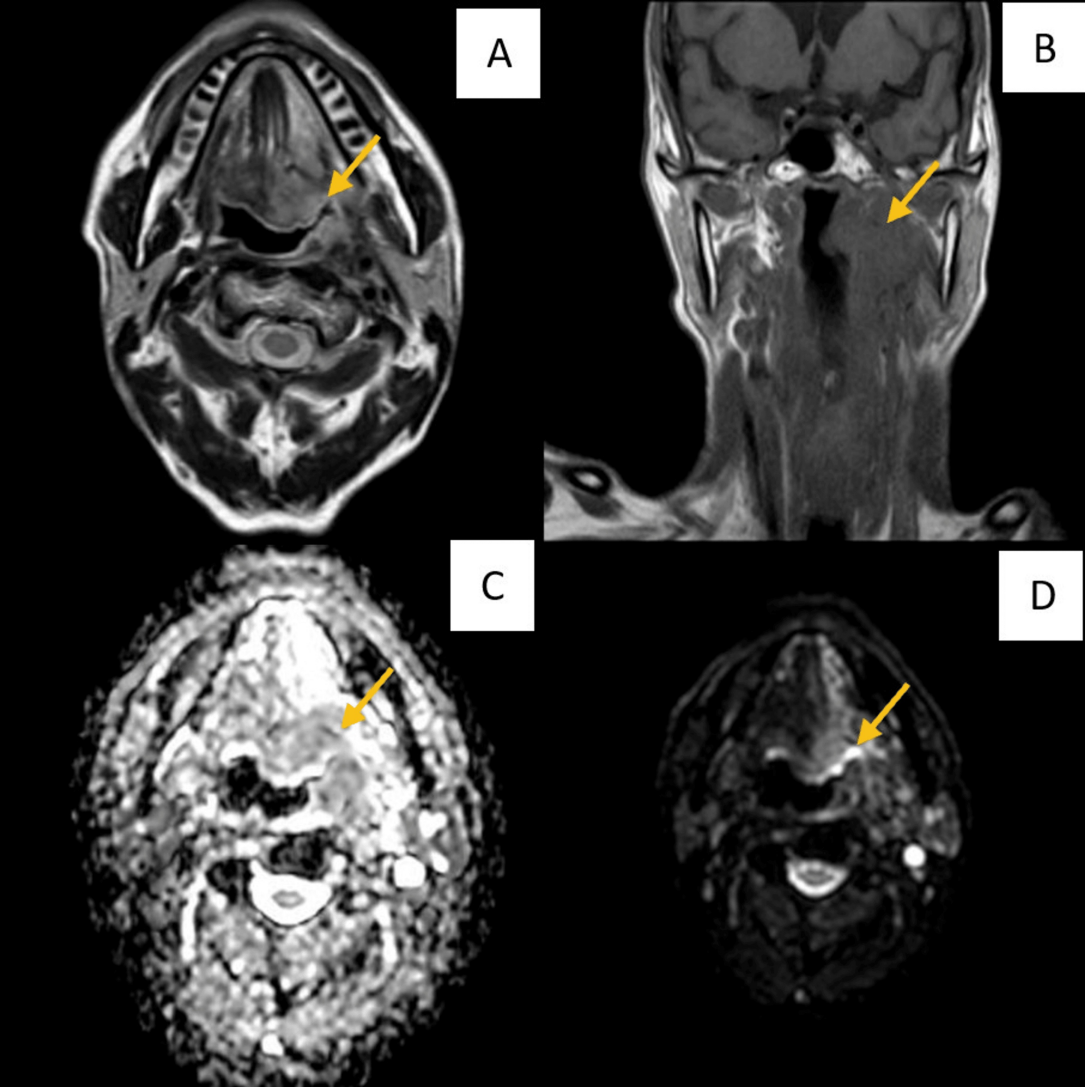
MRI images of a 57-year-old male, showing an ill-defined, diffusion restricting enhanced lesion noted in the left posterior two-third of the tongue and base of the tongue - likely malignant neoplastic etiology A: T2-weighted imaging MRI sequence showing an ill-defined T2 hyper-intense lesion seen in the left lateral aspect of the posterior two-third of the tongue and base of the tongue; B: T1-weighted imaging MRI sequence showing an ill-defined T1 iso-intense lesion seen in the left lateral aspect of the posterior two-third of the tongue and base of the tongue; C and D: Apparent diffusion coefficient (ADC) and DWI images showing diffusion restriction
